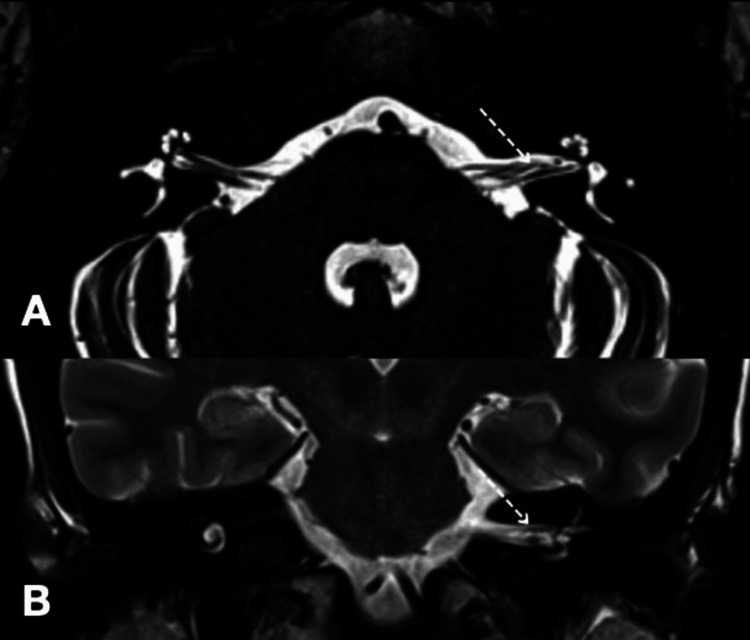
Magnetic resonance image analysis of Case 1 of constructive interference in steady state (CISS) sequence (A) and coronal T2 (B) showing a loop of the anterior inferior cerebellar artery (AICA) (dotted arrow) coursing between the facial nerve and vestibulocochlear nerve within the left internal auditory canal.
